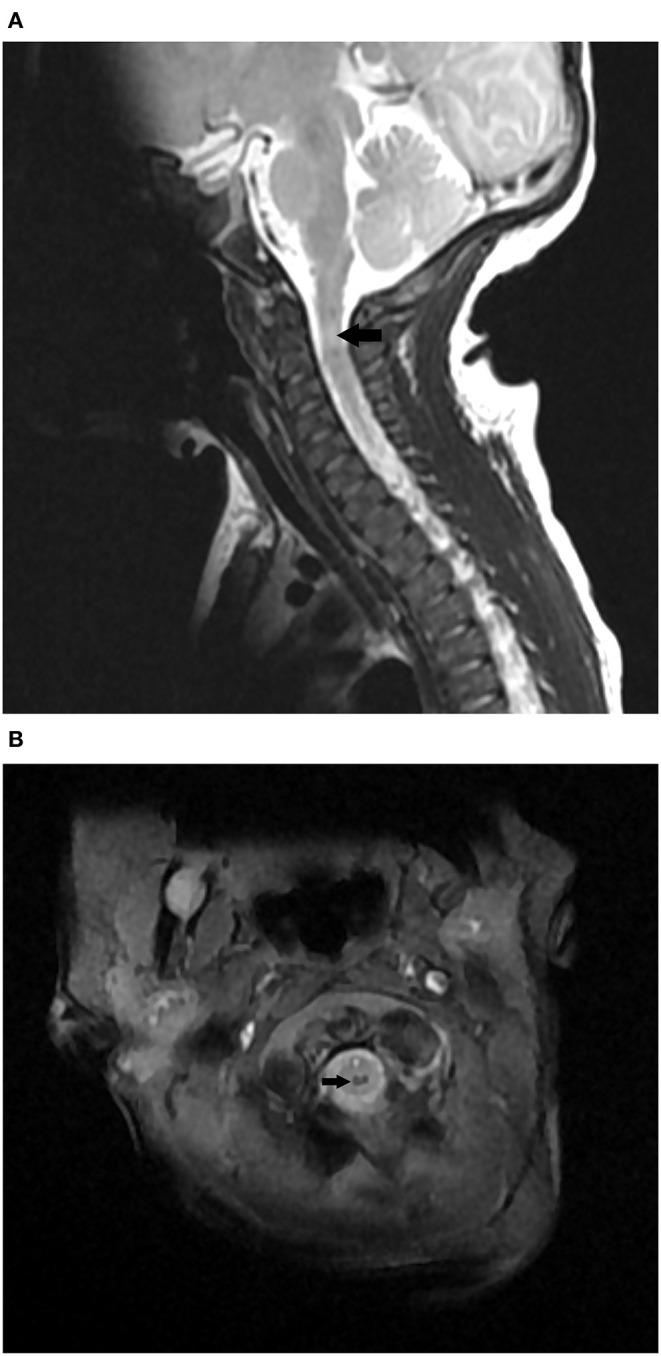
(A) MRI Sagittal T2 image showing mixed hypo- and hyper-intensity lesion over the cervical spinal cord at the C2 level. (B) MRI axial MERGE image showing hypo-intensity in spinal cord at the C2 level.
